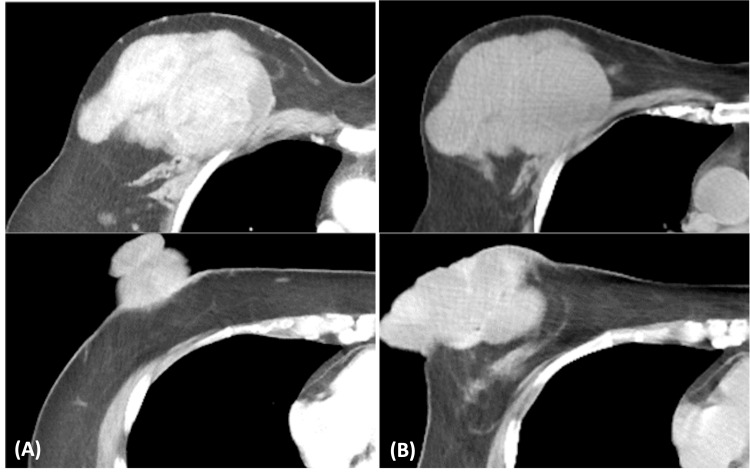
Computed tomography of the ruptured phyllodes tumor (A) Contrast-enhanced computed tomography (CT) findings at the initial examination findings demonstrated a 110×85 mm mass with contrast effects and calcification compressing the skin and pectoralis major muscle and a 40×35 mm mass erupting from the paramammary nipple. (B) CT findings three days after the initial examination showed a 105×75 mm mass with contrast effects and a 100×45 mm mass erupting from the paramammary nipple.
